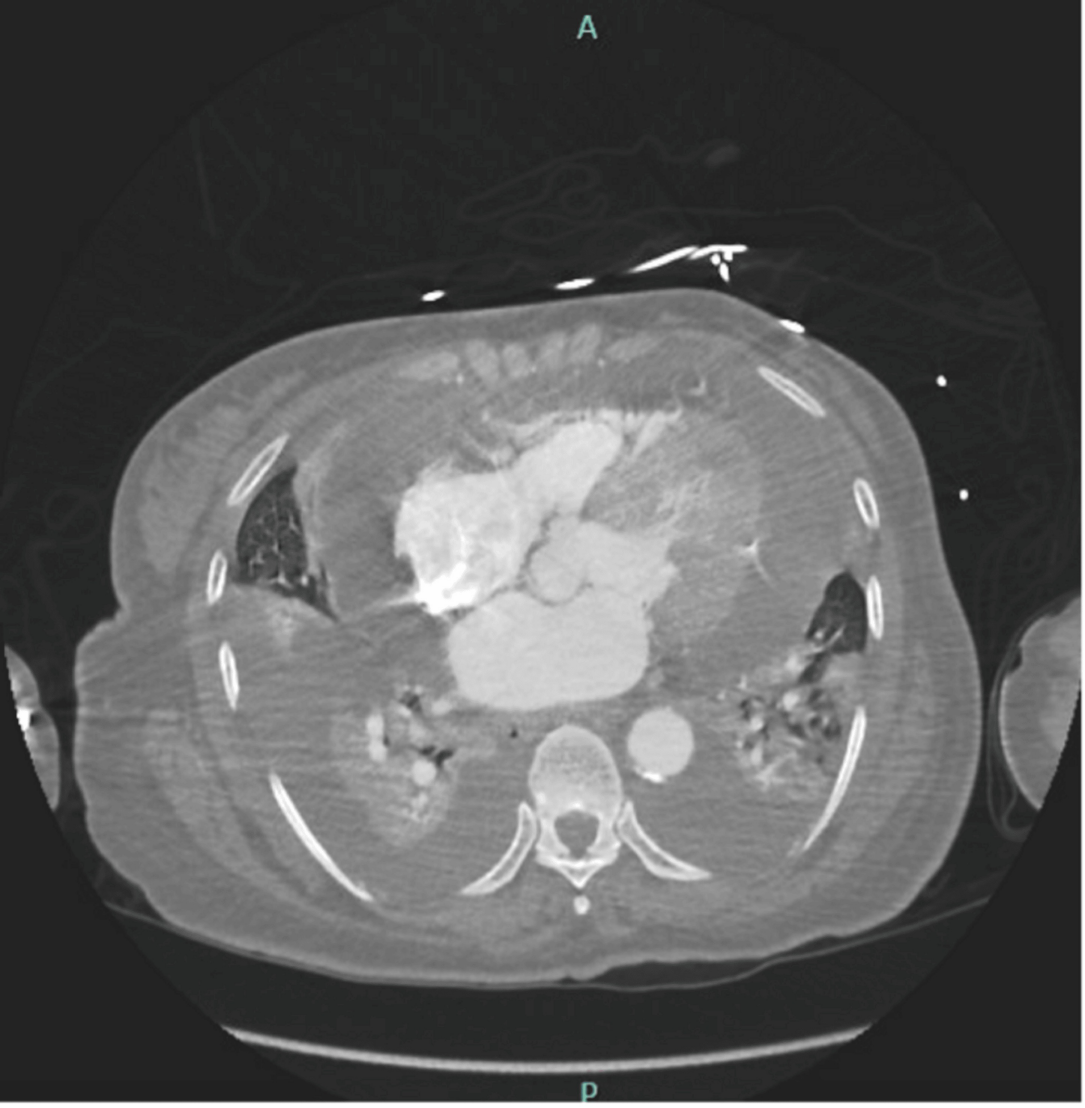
Computed tomography angiography of the chest showing bilateral pleural effusion and pericardial effusion.
